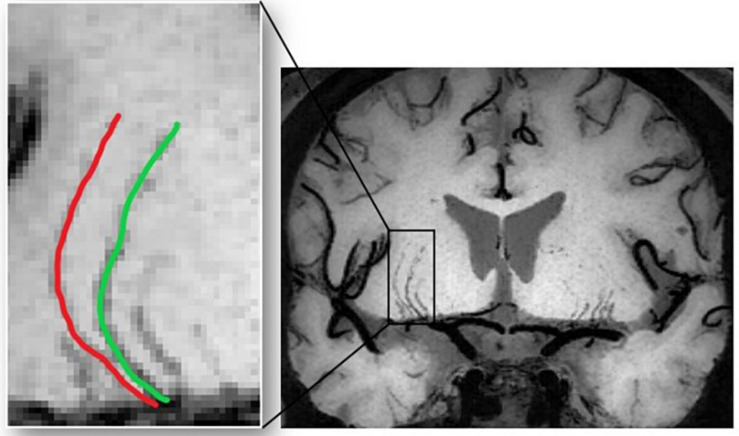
The images are coronal 10-mm thin-slice minimum intensity projections of 3D black blood MRI for presentation. LSAs were manually delineated on the images and morphological measurements were calculated accordingly.
